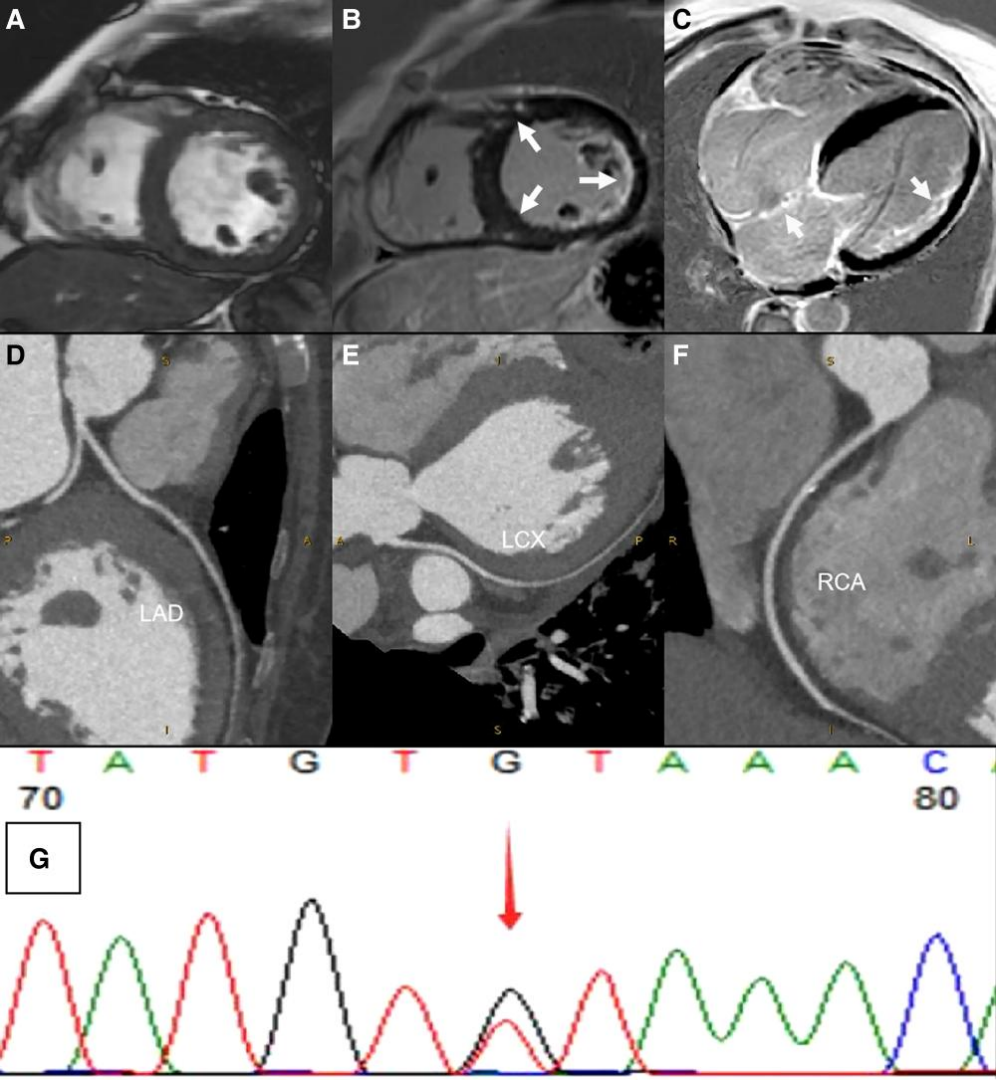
(*A*) Cardiac magnetic resonance imaging short-axis sagittal view showing a moderately dilated left atrium and ventricle, with mild inter-ventricular septum thickening (15 mm). (*B* and *C*) Late gadolinium enhancement at 30 min post-contrast showed a region of enhancement at the right ventricular insertion points, sub-endocardium of the left ventricular lateral wall, trabeculae, and inter-atrial septum (arrow). (*D–F*) Coronary computed tomography angiography showed the left anterior descending artery, left circumflex coronary artery, and right coronary artery with no significant stenosis. (*G*) Whole-exome sequencing identified the mutation (anoctamin 5 c.1301G>T).
